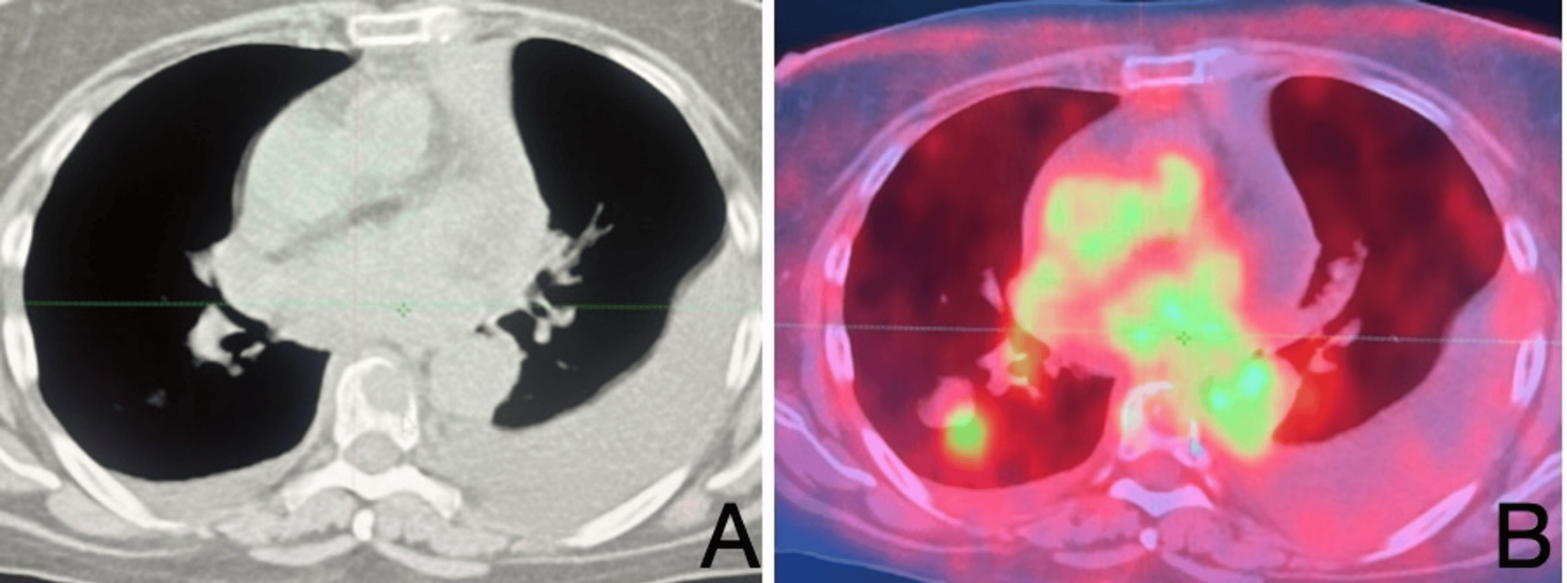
Patient 1: (A) CT scan showing a malignant mass infiltrating the thoracic vertebral body. (B) PET-CT demonstrates intense hypermetabolic activity in the same lesion, consistent with metastatic thoracic spine involvement. CT: computed tomography; PET-CT: positron emission tomography-computed tomography.
